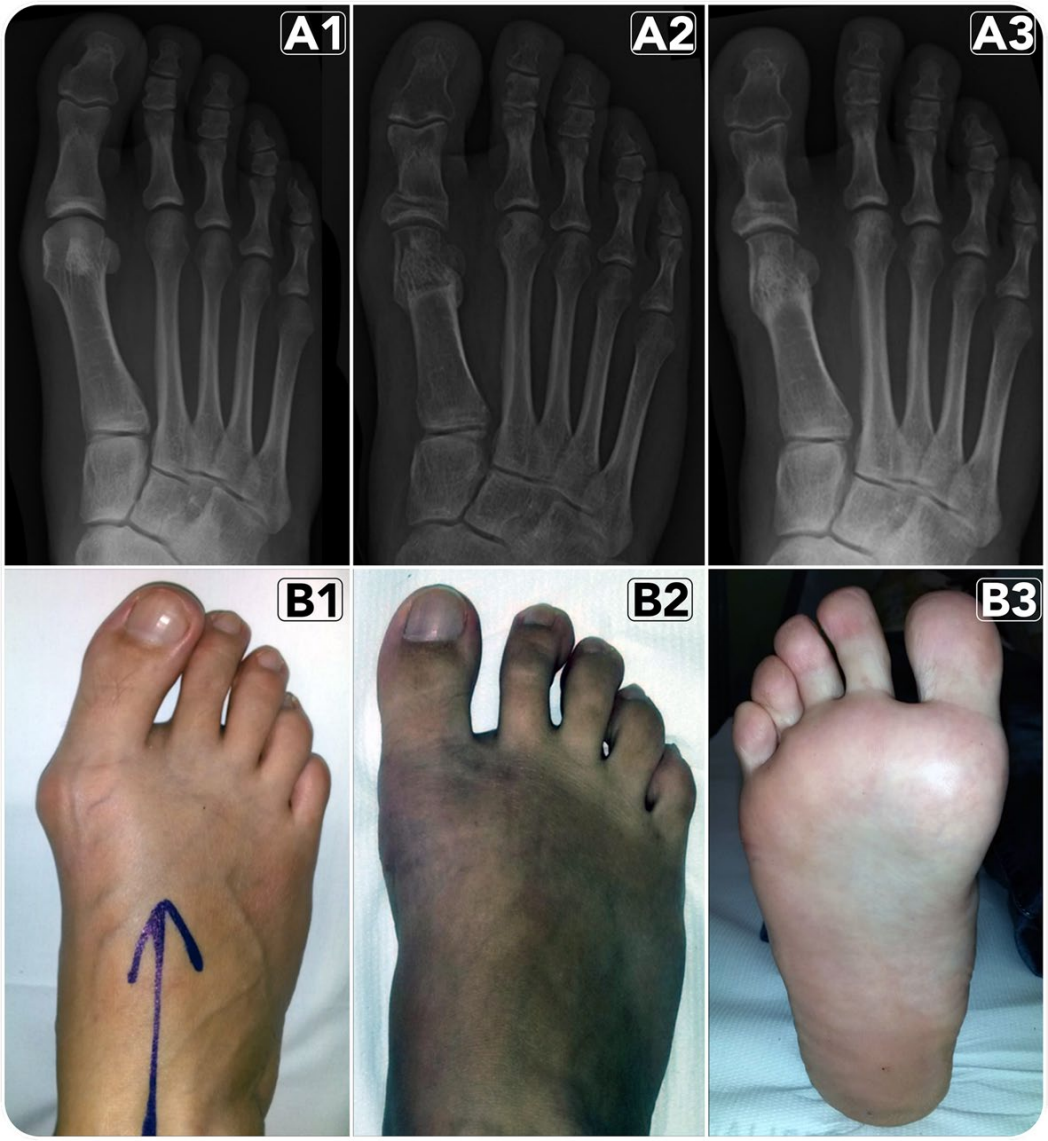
A 39-year-old woman with right mild HV after having undergone percutaneous Reverdin-Isham osteotomy, lateral release and Akin osteotomy for HV correction: (A) antero-posterior radiographic images at preoperative period (1), 3-month follow-up (2) and 6-month follow-up (e). (B) Clinical images at preoperative period (1) and at 6-month follow-up (1–2)
